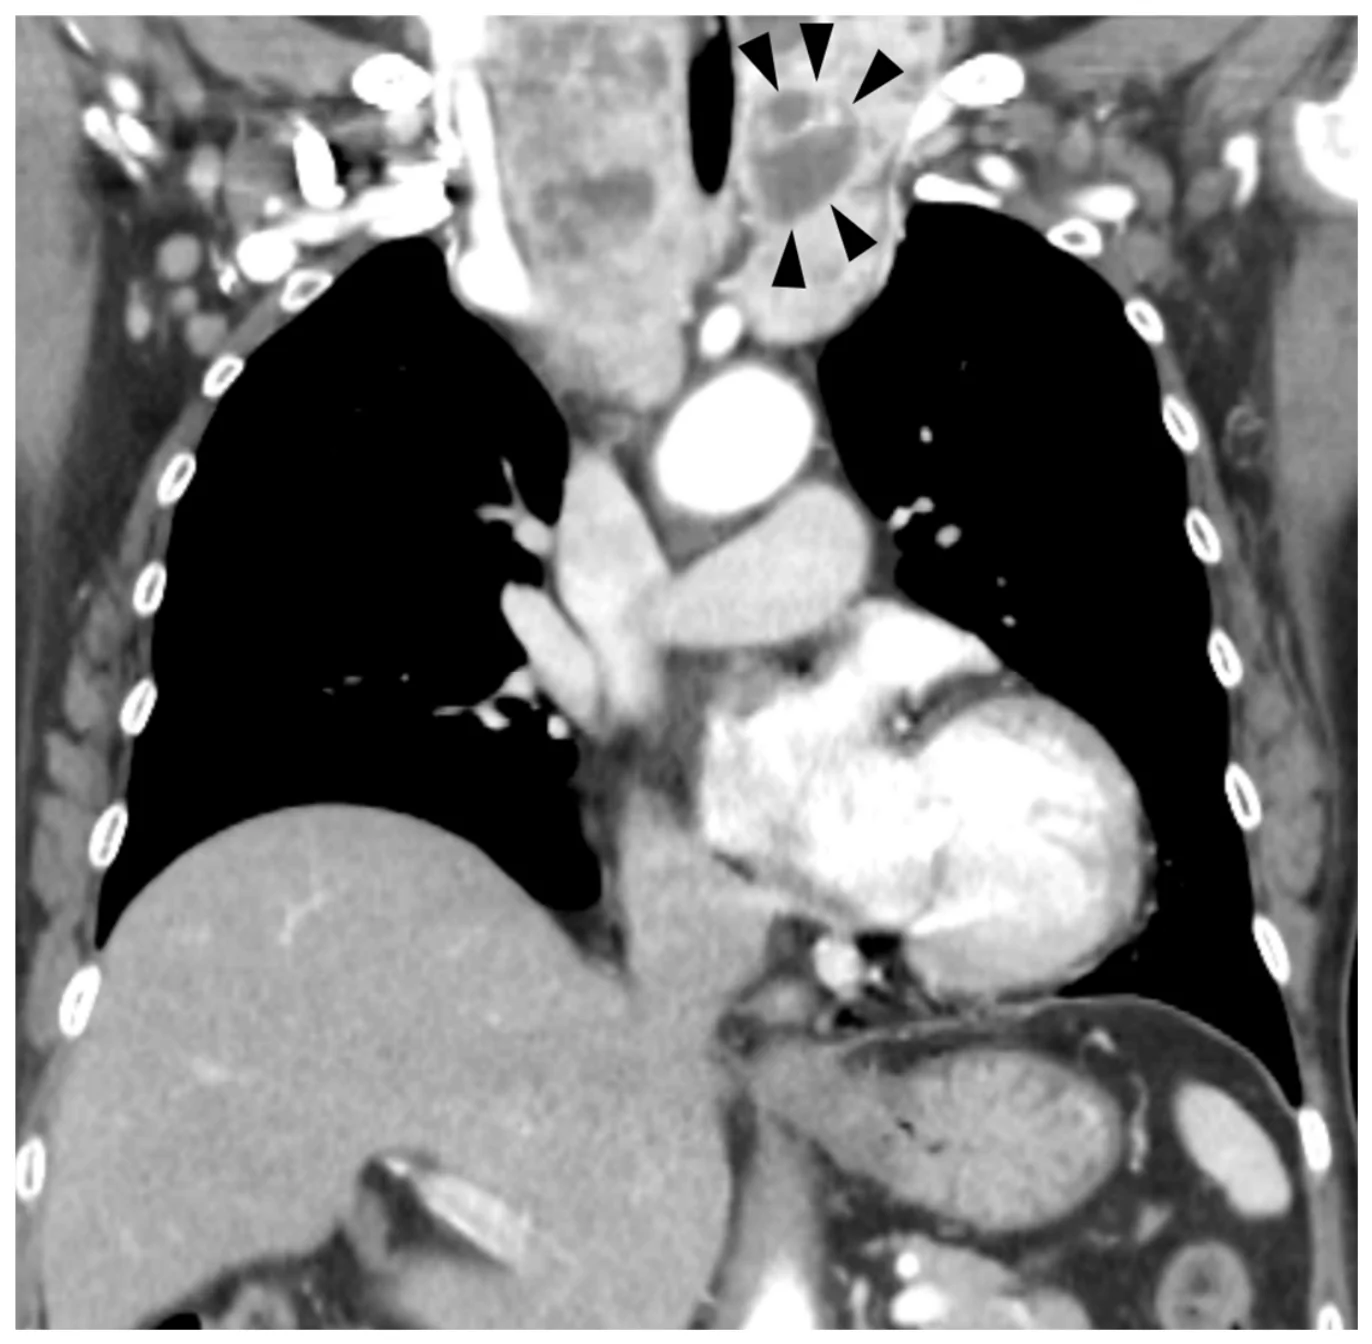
A case of multinodular goiter in a 66-year-old woman. Note multiple nodules in the enlarged thyroid glands. Some nodules in the left thyroid gland show sharp margination (arrowheads). This is a characteristic finding of multinodular goiter compared to shock thyroid on enhanced chest CT. TFTs were normal in this patient.
